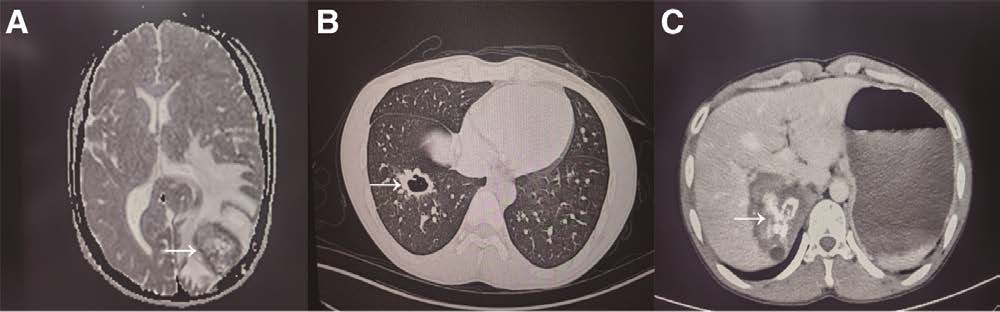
(A) MRI shows hypoperfusion within the lesion and hyperperfusion at the margin, suggesting alveolar echinococcosis (AE) (horizontal white arrow, parameters: T2-TSE; TR/TE, 3500/95 ms; slice thickness, 5 mm). (B) CT shows a lesion in the anterior basal segment of the lower lobe of the right lung with cavity formation, suggesting hepatic multilocular echinococcosis lung M (horizontal white arrow, parameters: tube voltage, 120 kV; slice thickness, 1.0 mm). (C) CT shows hepatic multilocular echinococcosis (horizontal white arrow, parameters: tube voltage, 120 kV; slice thickness, 5 mm). CT = computed tomography, MRI = magnetic resonance imaging.
